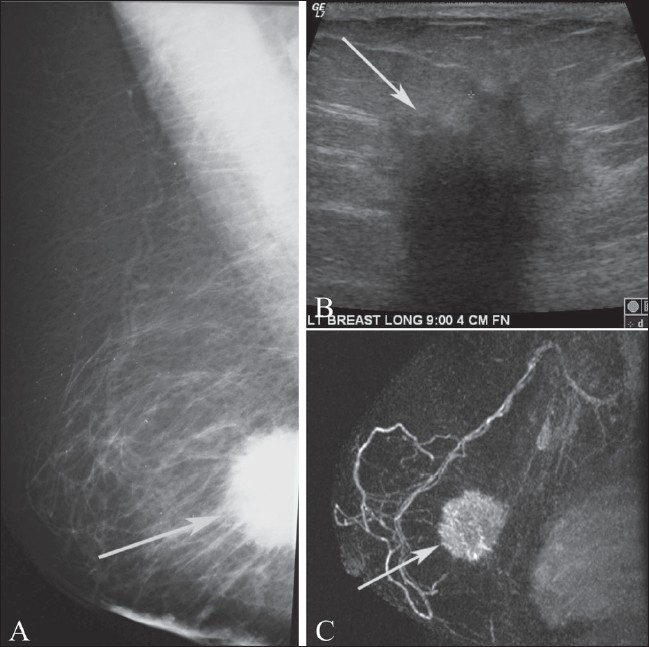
(Mediolateral oblique mammographic view (A) of a patient who complained of shrinking breast size. A spiculated mass (arrow) is seen in the posterior one-third of the breast, which was incompletely evaluated by mammography. The USG (B) demonstrates an angular and spiculated lesion (arrow) with posterior acoustic shadowing, which hinders evaluation of the fascia and muscle. Only the MIP MRI image (C) clearly demonstrates that although the retroglandular fat is obliterated, there is no chest wall invasion (arrow)
